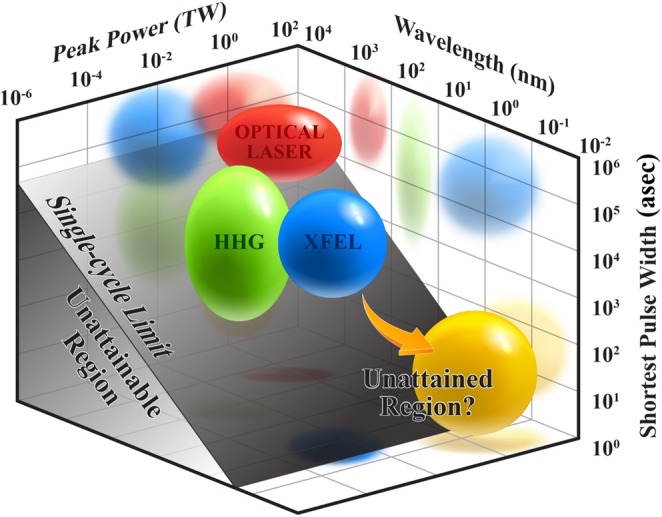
Current ultrafast photon sources in the view of pulse duration, wavelength and pulse power. HHG: high-order harmonic generation; XFEL: X-ray free-electron laser.
